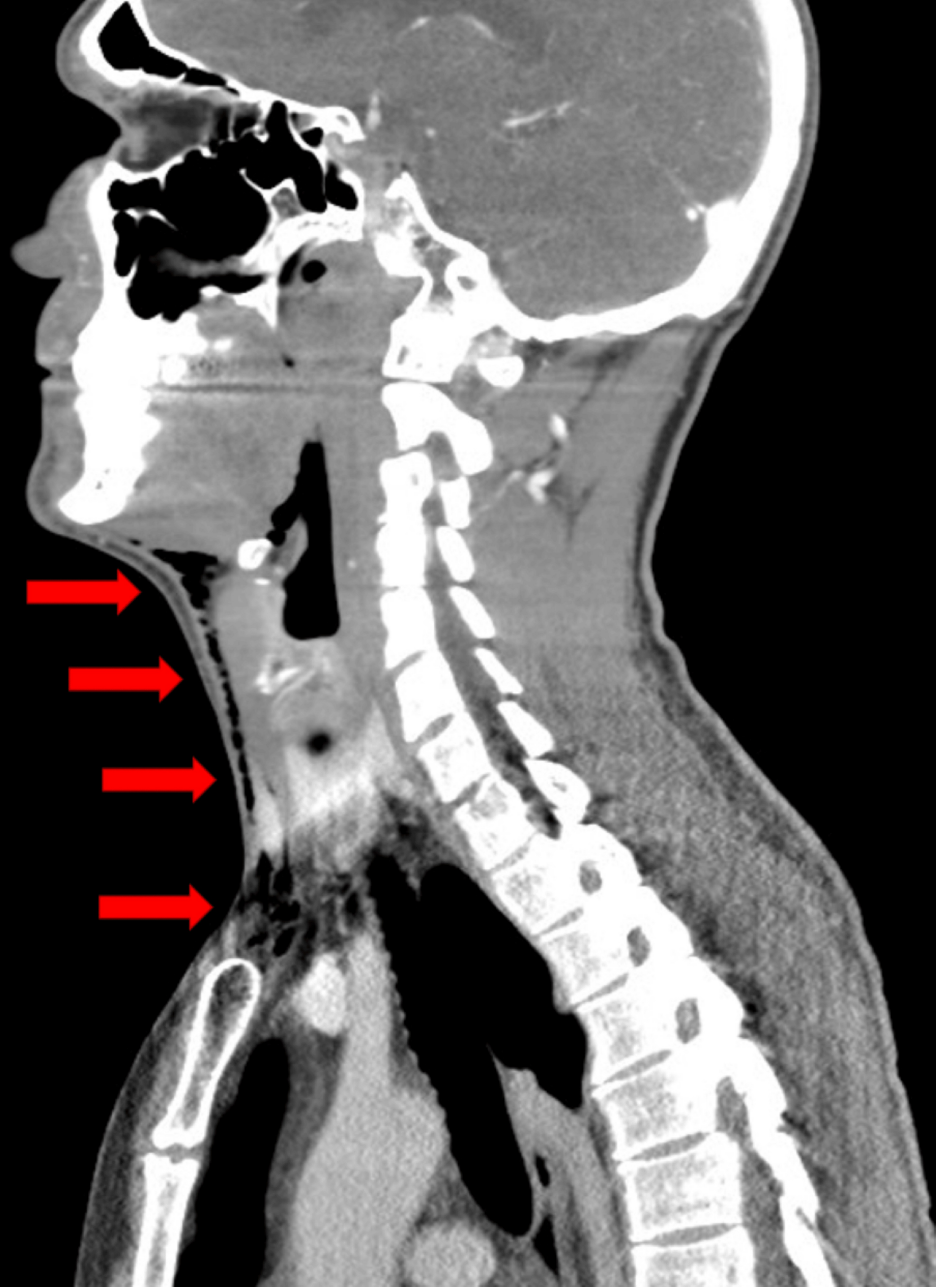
Computed Tomography: Sagittal View Red arrows indicate areas of subcutaneous emphysema.
